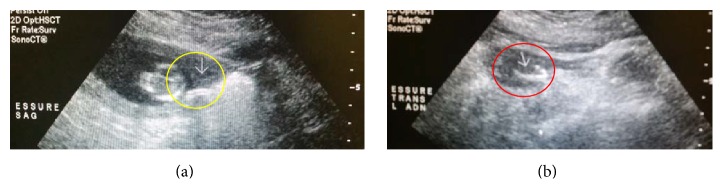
Patient number 2, pelvic sonogram on 4/2/15: showing right Essure inserts, sagittal view (yellow circle), and left Essure insert, transverse view (red circle).
